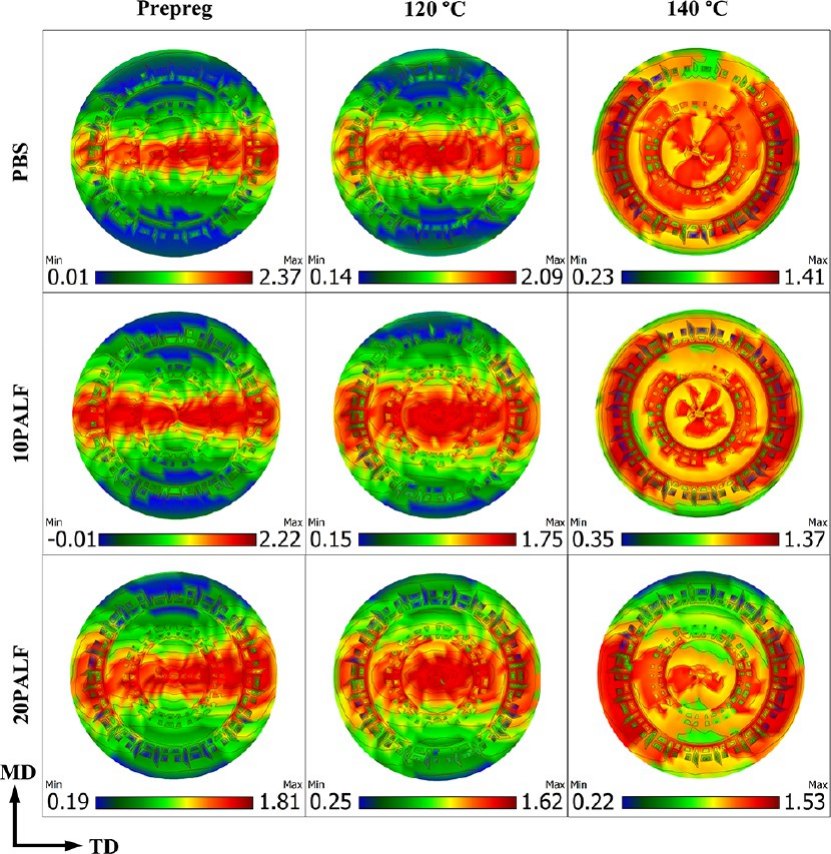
X-ray pole figures for the (110) plane of PBS and PBS/PALF
